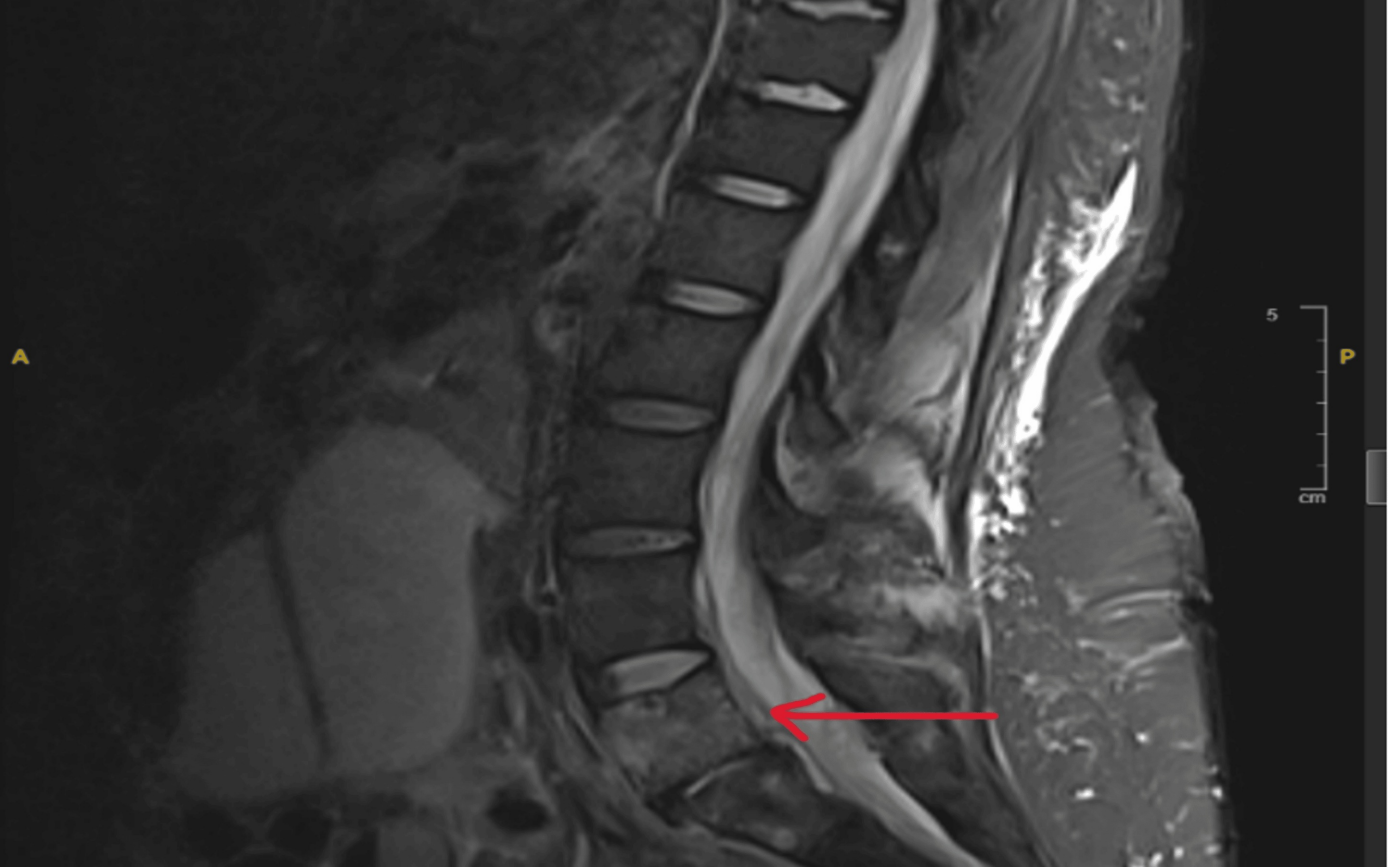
MRI of the spine showing L5-S1 discitis concerning for potential osteomyelitis.
